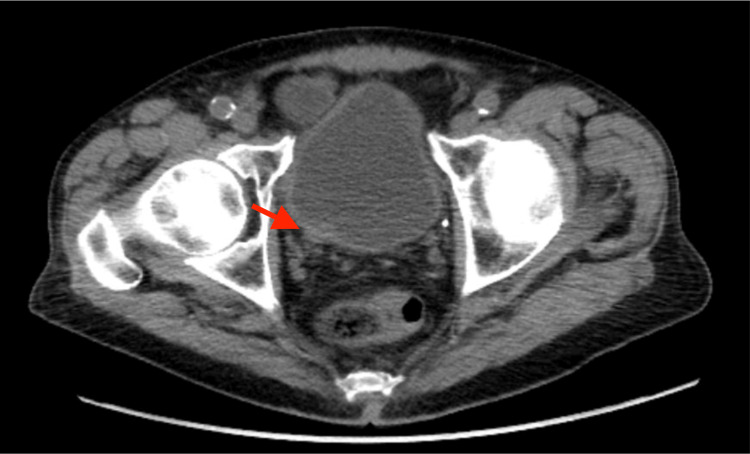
Image from CT pelvis depicting lesion at the ureteral insertion of a bladder CT: computed tomography
